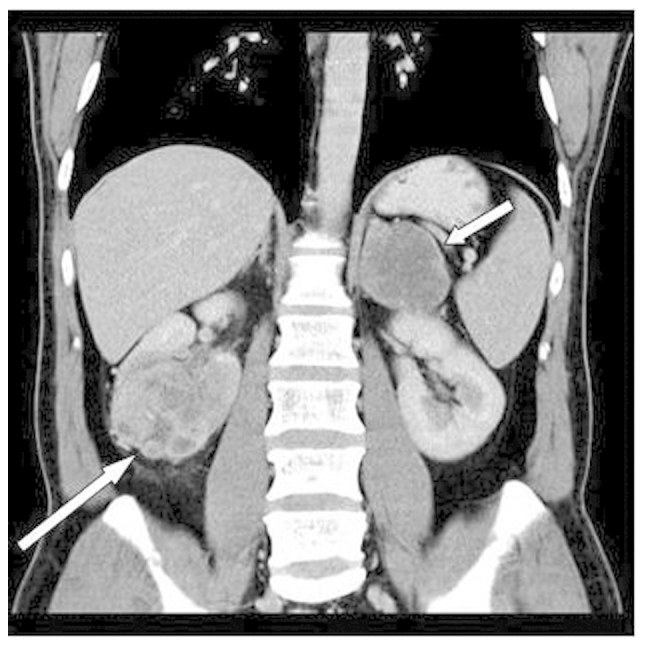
Computed tomography imaging of the coronal reconstruction indicating the right renal mass (long arrow) and the left adrenal metastatic mass (short arrow).
